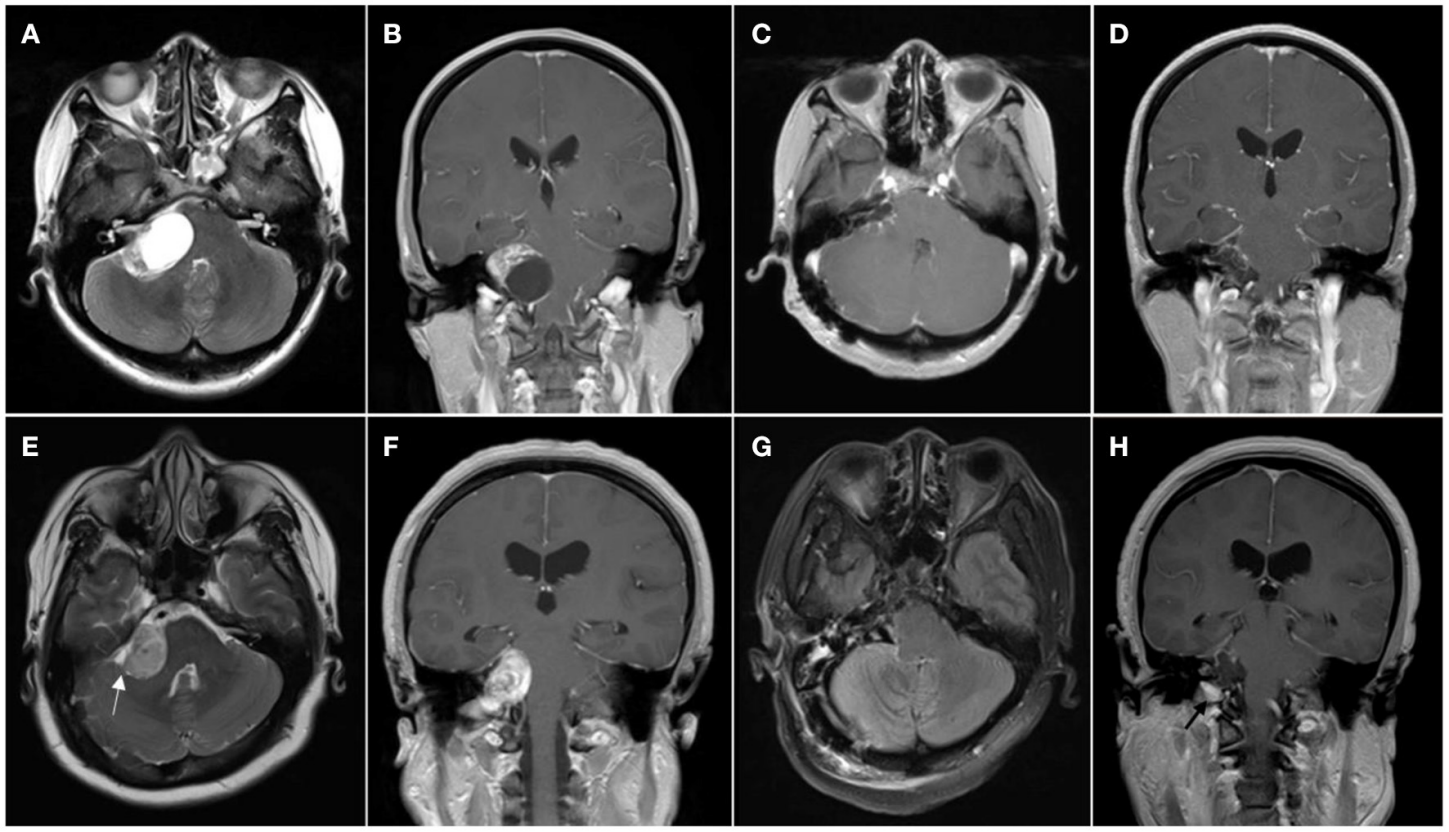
Patient with cystic vestibular schwannoma. (A,B) Preoperative MR images showing cystic vestibular schwannoma; (C,D) Postoperative MR images showing resection of the tumor. (E–H) Patient with solid vestibular schwannoma; (E,F) Preoperative MR images showing solid vestibular schwannoma, cerebrospinal fluid (CSF) cleft sign surrounding the tumor is marked with the white arrowhead; and (G,H) Postoperative MR images showing resection of the tumor and left tumor remnant is marked with the black arrowhead.
